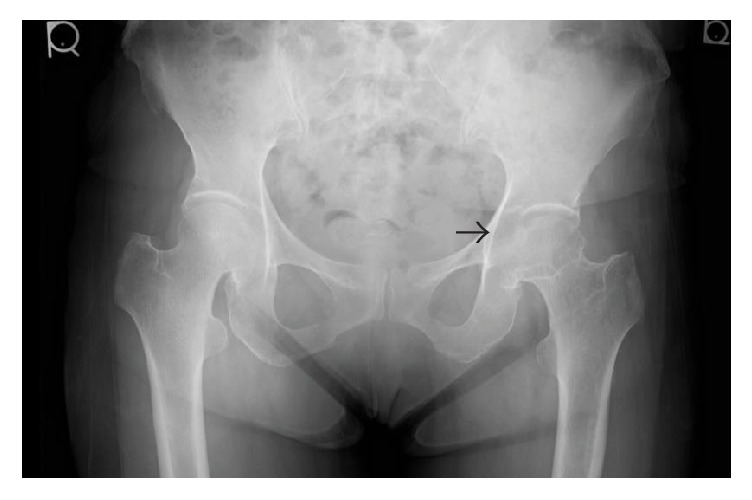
Anterior-posterior radiograph of the hip. Enlargement of the acetabular fossa is shown (black arrow).
